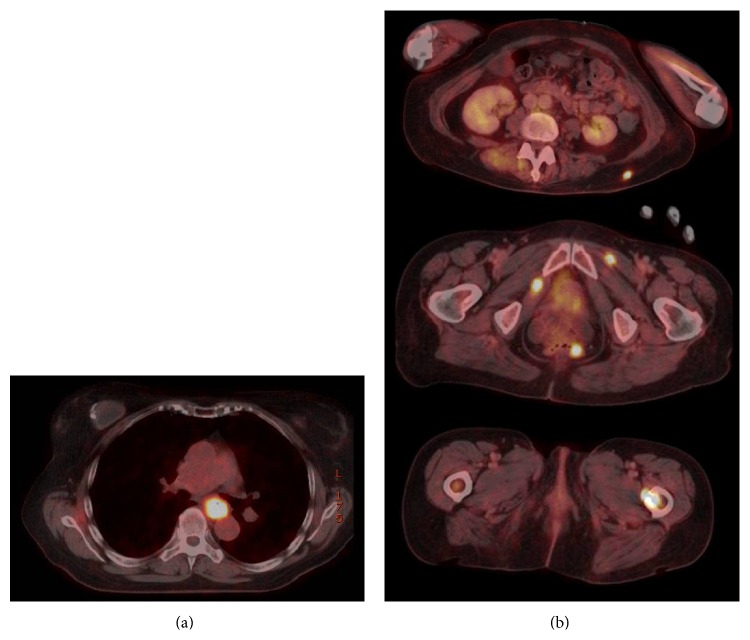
(a) Combined PET-CT scan with 283 Mbq F 18 FDG and computed tomography with iodine biased contrast agent. Area of screening: scull base till proximal femur. It reveals the large soft tissue mass with a significant increase in metabolic activity (SUV max 22.9) which surrounds the dorsal part of the thoracic esophagus. (b) Multiple cutaneous, muscular, and osseous metastases (SUV max 22.9) in the entire range of screening.
